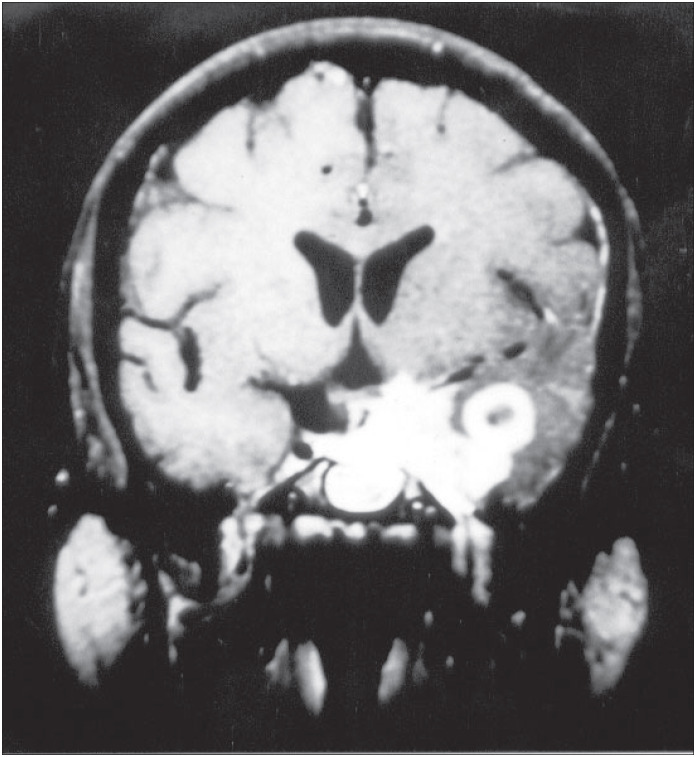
Coronal magnetic resonance imaging of the brain in the T1-weighted series after gadolinium injection, showing a lesion over the left temporal lobe in its mesial aspect and infiltrating the left cavernous sinus, with high signal.
